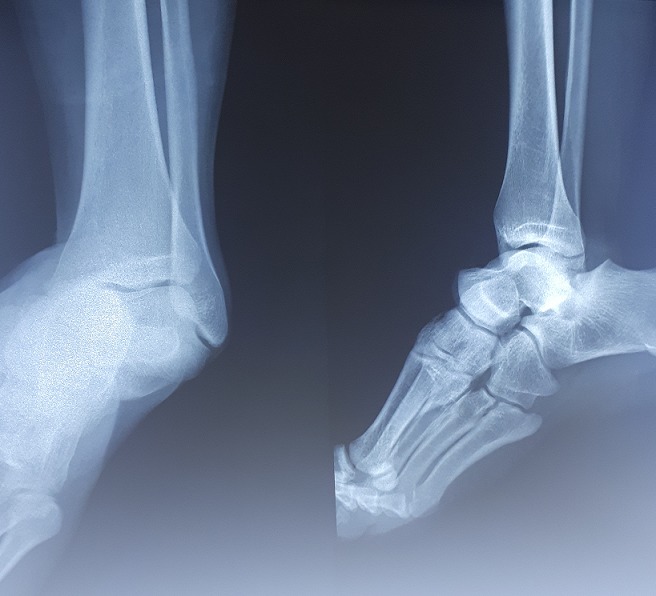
Radiographie standard du pied et de la cheville de face et de profil confirme la luxation sous talienne interne
